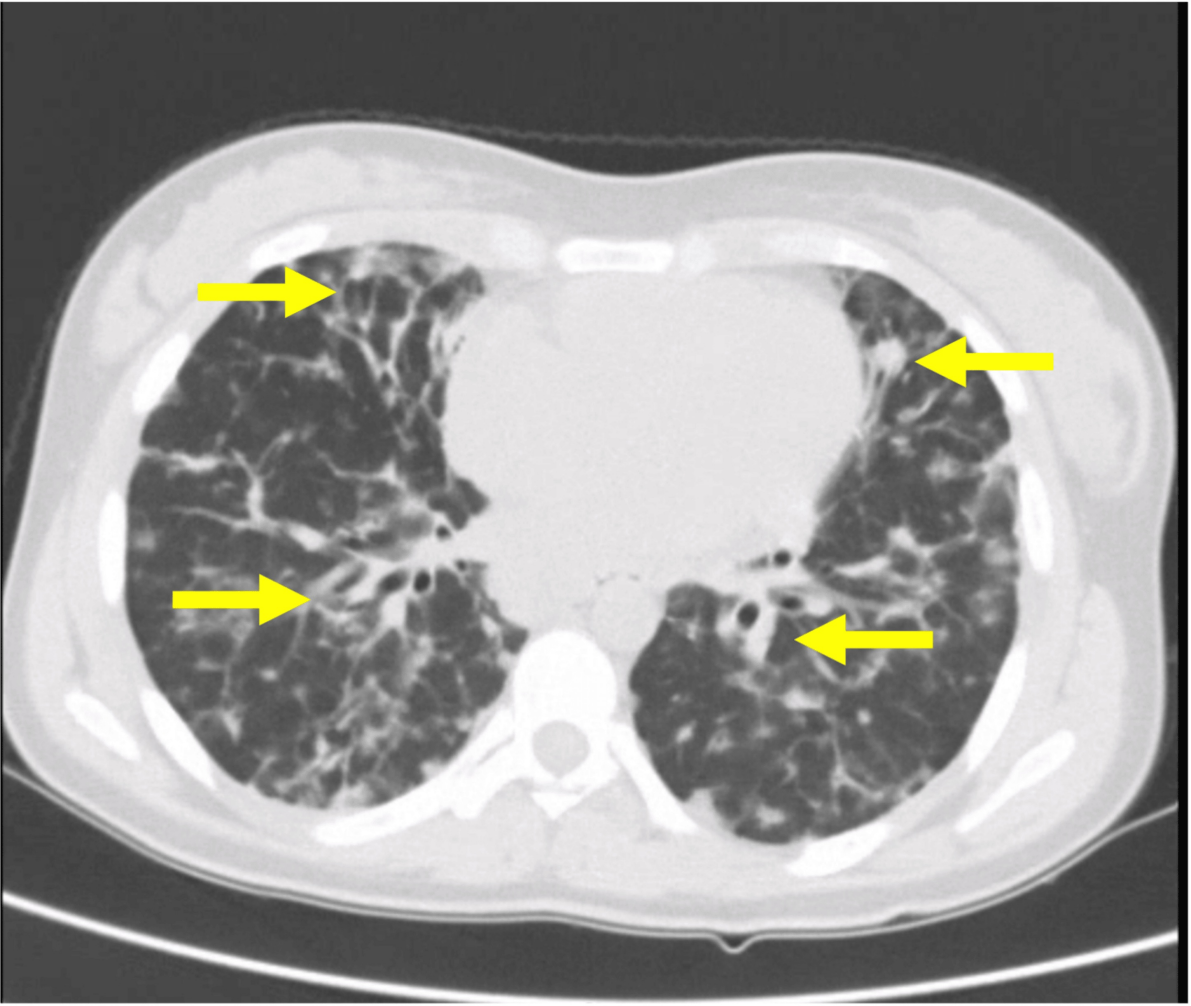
Axial plane of chest computed tomography Multiple centrilobular nodules, areas of ground-glass opacities, and subpleural consolidations, predominantly in basal lung segments (arrows)
